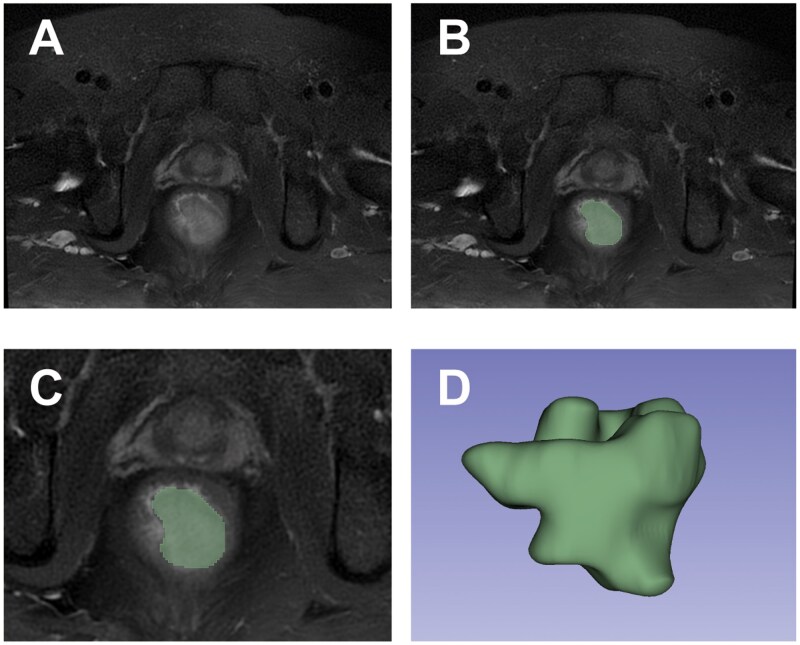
Tumor segmentation and 3D reconstruction using 3D-slicer software. (A) Axial T2-weighted MRI image of a colorectal tumor, showing the tumor without any annotations. (B) Delineation of the ROI on a single MRI slice, with the tumor’s boundary marked for radiomics feature extraction. (C) Magnified view of the segmented ROI from panel B, highlighting the tumor’s precise boundary. (D) 3D reconstruction of the tumor volume based on multi-slice ROI annotations, offering a visual representation of the tumor’s spatial morphology. Tumor segmentation and 3D reconstruction were performed using the 3D-Slicer software (www.slicer.org) to facilitate the extraction of radiomics features from the MRI data. MRI, magnetic resonance imaging; ROI, region of interest.
